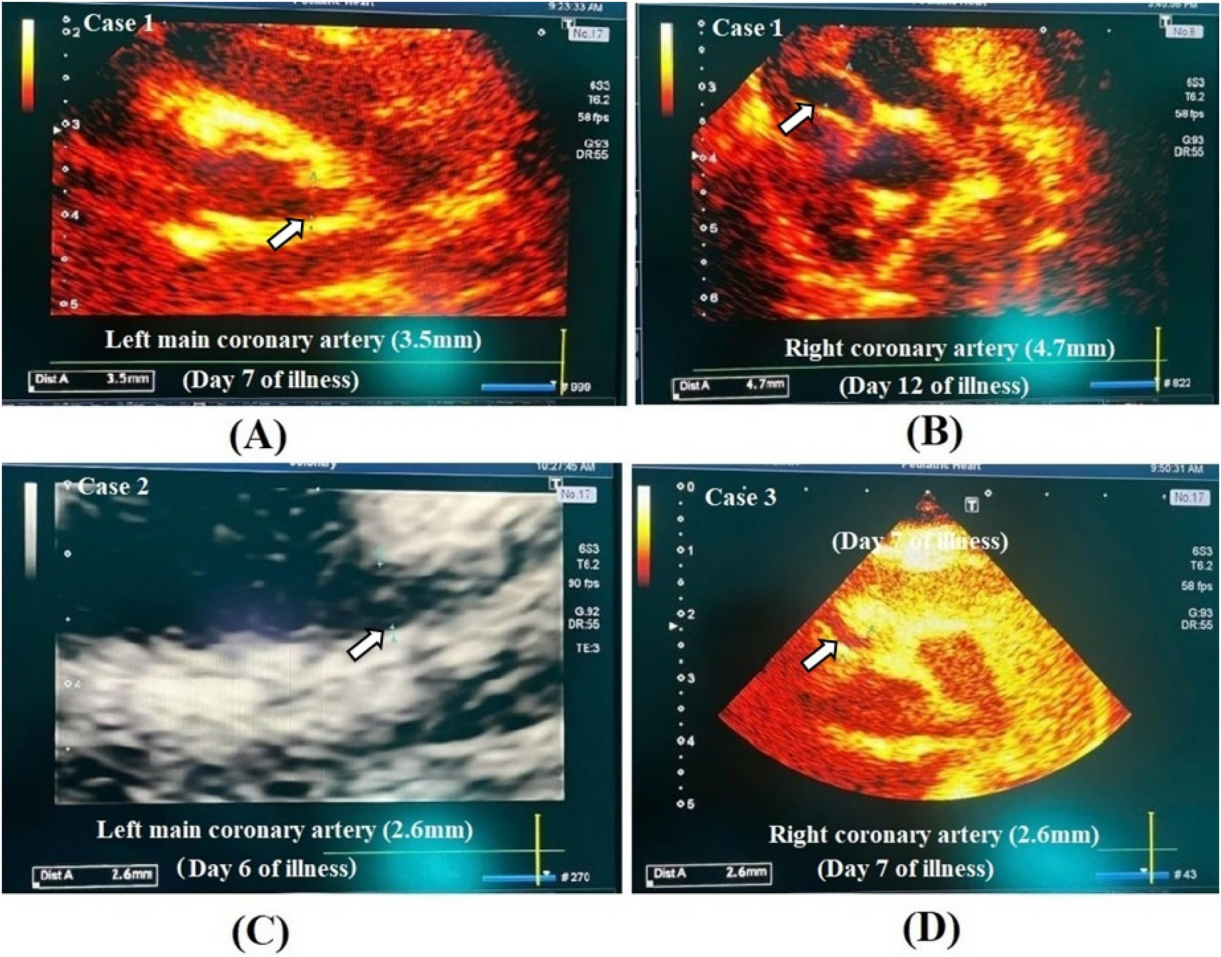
Echocardiographic image of coronary artery lesion in case 1 (A,B), case 2 (C) and case 3 (D).
